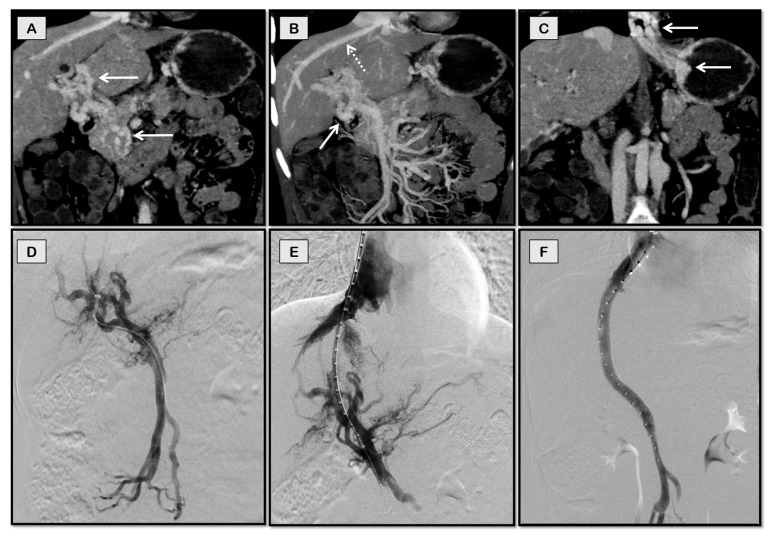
TIPS through a collateral vein for recurrent variceal bleeding in a 17-year-old girl with chronic non-cirrhotic portal vein thrombosis. She had undergone a splenectomy at the age of 6 years and was not a candidate for Rex shunt. Coronal-oblique CT images (A–C) showing portal cavernoma (arrows in A), dominant collateral vein (dashed arrow in B) in the same plane as middle hepatic vein (solid arrow in B), and esophageal and gastric varices (arrows in C). The collateral vein was accessed through a jugular route (D) and stented (E) with flow restoration and disappearance of collaterals (F).
